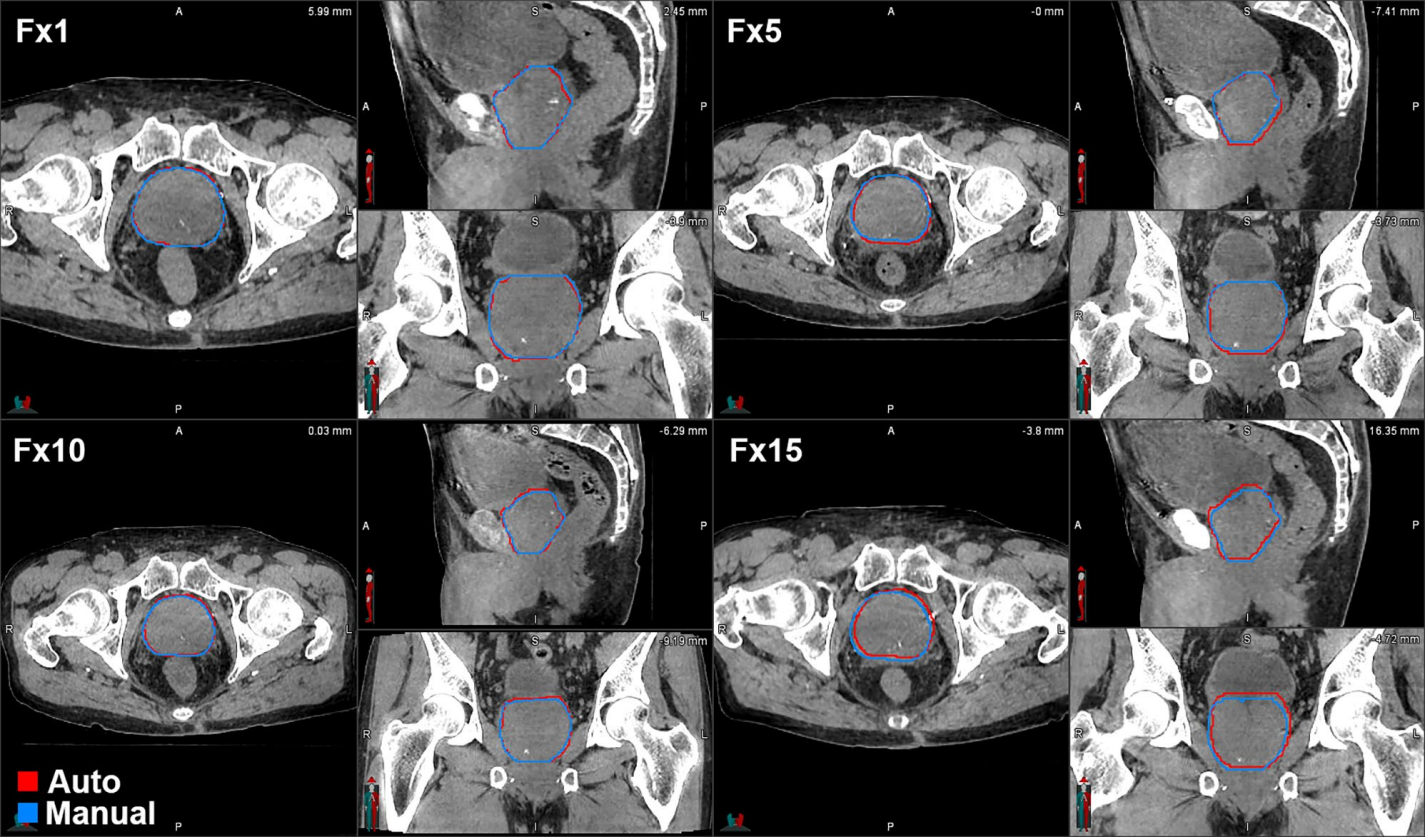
Example CBCT images of a prostate patient for different fractions during radiotherapy treatment. Automated prostate contours are shown in red and manual contours in blue. The images shown here were reconstructed using iterative reconstruction algorithm.
